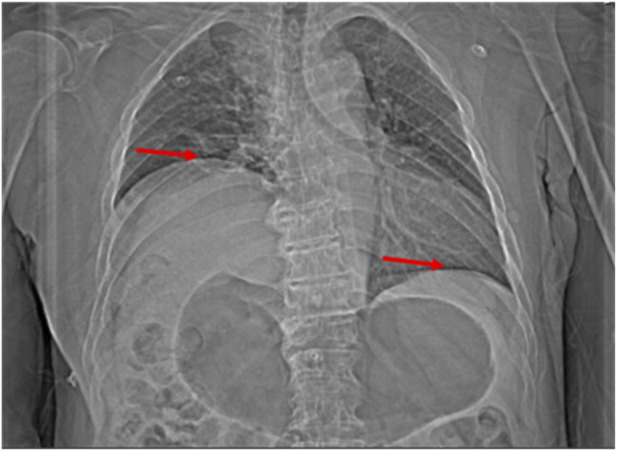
Chest CT (scout view) on postoperative day 42 showing a continued elevation of the right hemidiaphragm compared to earlier scans.
